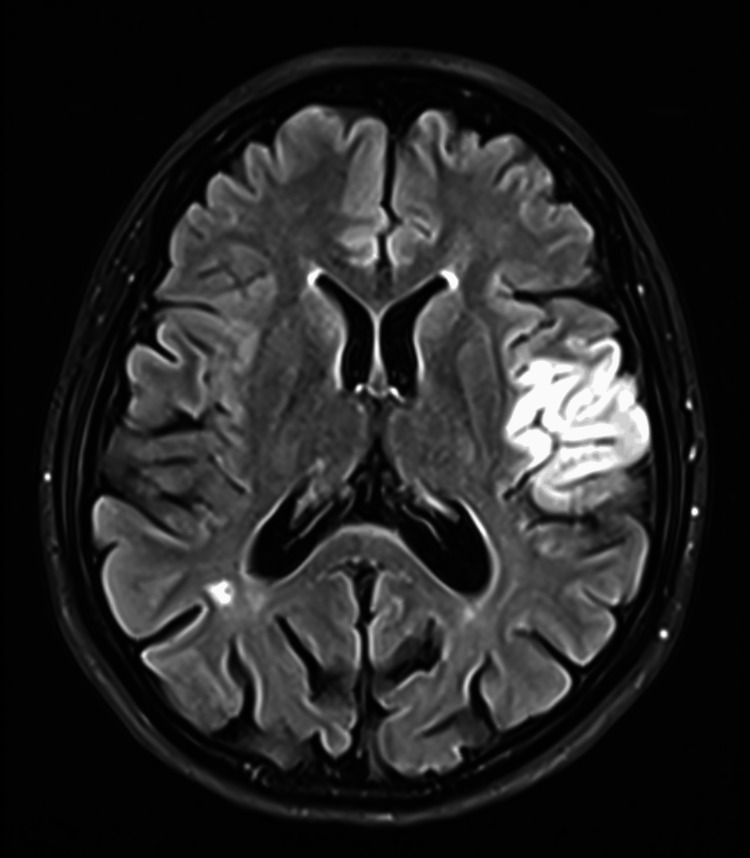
Axial magnetic resonance imaging (Flaire) Axial magnetic resonance (Flaire) shows the acute ischemic infarct in the territory of the left middle cerebral artery (MCA) involving the insula and frontoparietal lobe extending to the pre- and post-central gyri.
